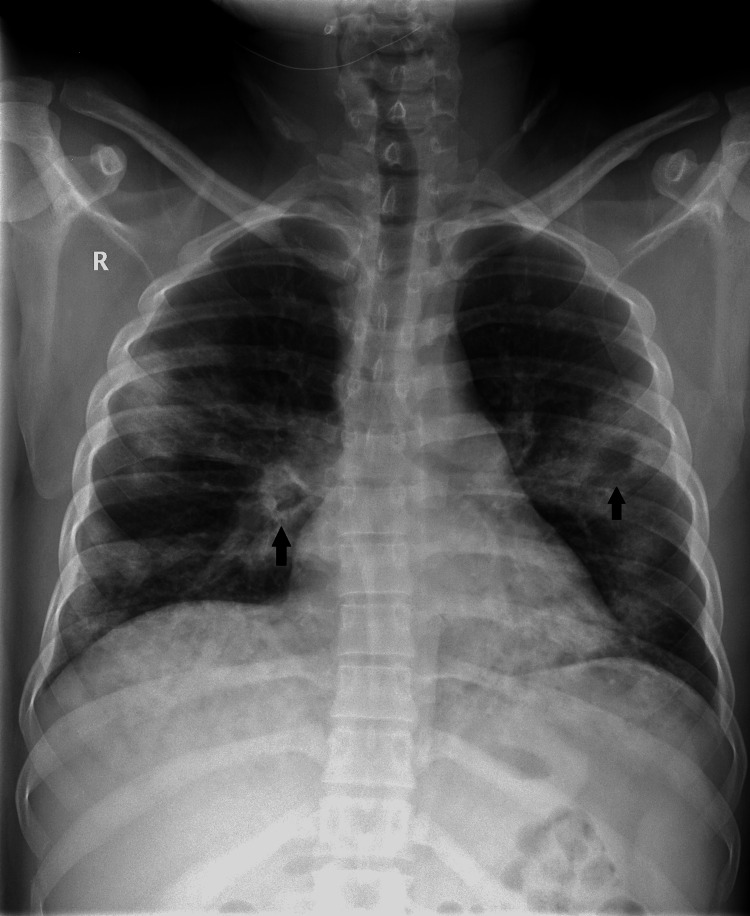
Chest x-ray shows bilateral parahilar and peripheral-based opacities, cavity-like radiolucent lesions (black arrows).
